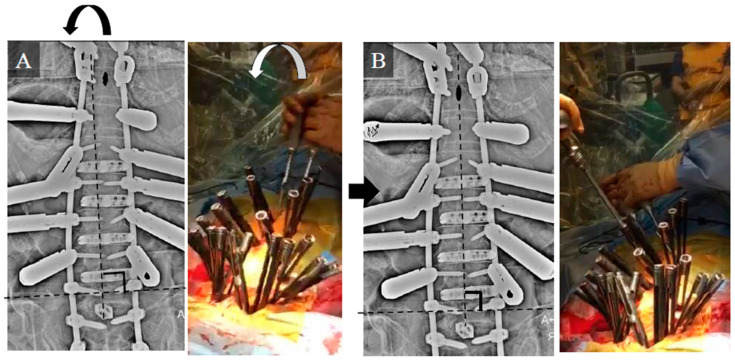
Intraoperative radiograph and photograph of the RR technique. (A) Intraoperative radiograph and photograph before RR. (B) Intraoperative radiograph and photograph after RR. Black oval: spinous process of the UIV. RR: rod rotation technique; UIV: upper instrumented vertebra.
